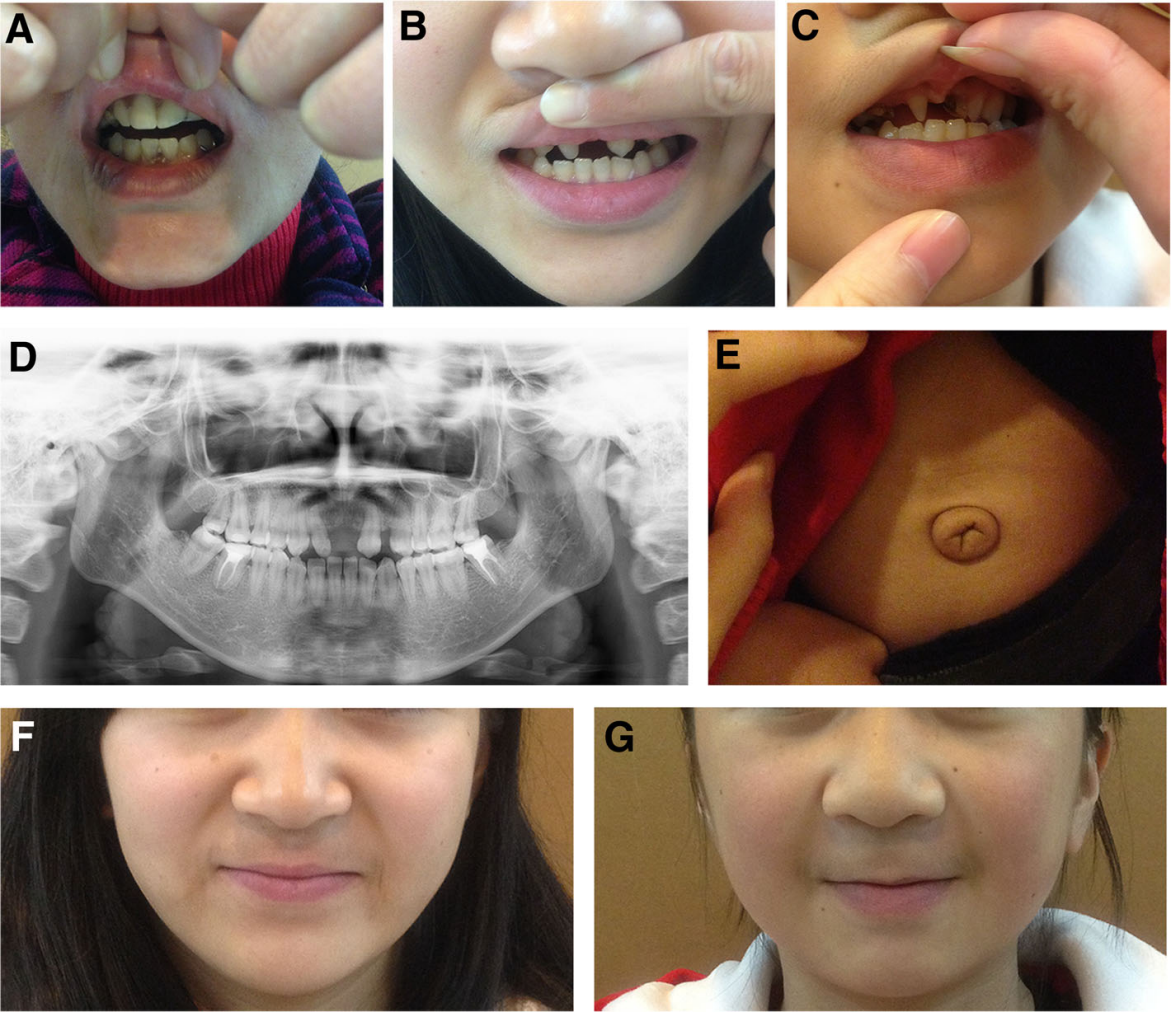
Systemic clinic features of affected family members with Axenfeld-Rieger syndrome. No microdontia was observed for I-2 because of a dental implant (a). Apparent microdontia for II-1 and II-2 (b and c). Dental panoramic radiography for II-1 (d), and II-2 had similar dental panoramic radiography. Umbilical defect (redundant periumbilical skin) for II-2 (e). All three patients exhibited the same sign when they underwent general physical examination. Broad nasal bridge of II-1 and II-2 (f and g)
